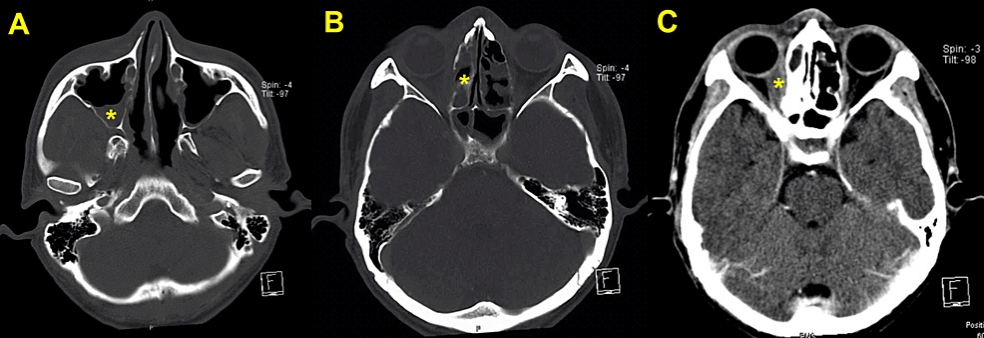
Paranasal CT scan of the patient (A) Mucoperiosteal thickening and air-fluid level in the right maxillary sinus (asterisk). (B) Mucoperiosteal thickening of right ethmoid sinuses (asterisk). (C) Diffuse haziness of extraconal and medial side of intraconal fat of right orbit (asterisk) CT: computed tomography
